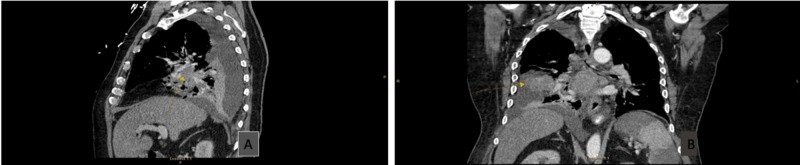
CT chest A: sagittal view; B: coronal view; Both show mass-like consolidation in the posterior right middle lobe with abnormal enhancement, suspicious for neoplasm (arrows)
